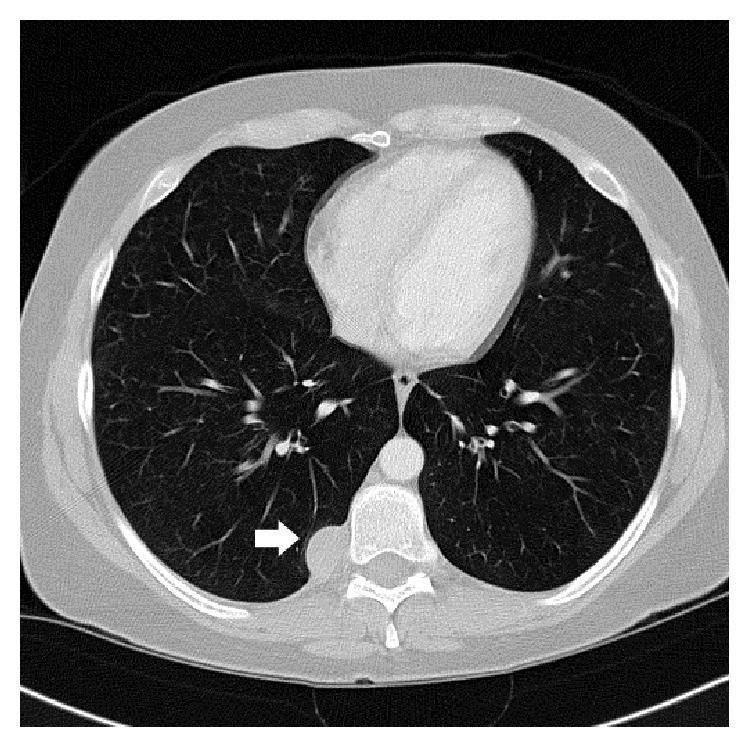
CT scan with contrast demonstrates a 2.0 cm posterior mediastinal mass abutting the right T9 rib and vertebral body (arrow).
